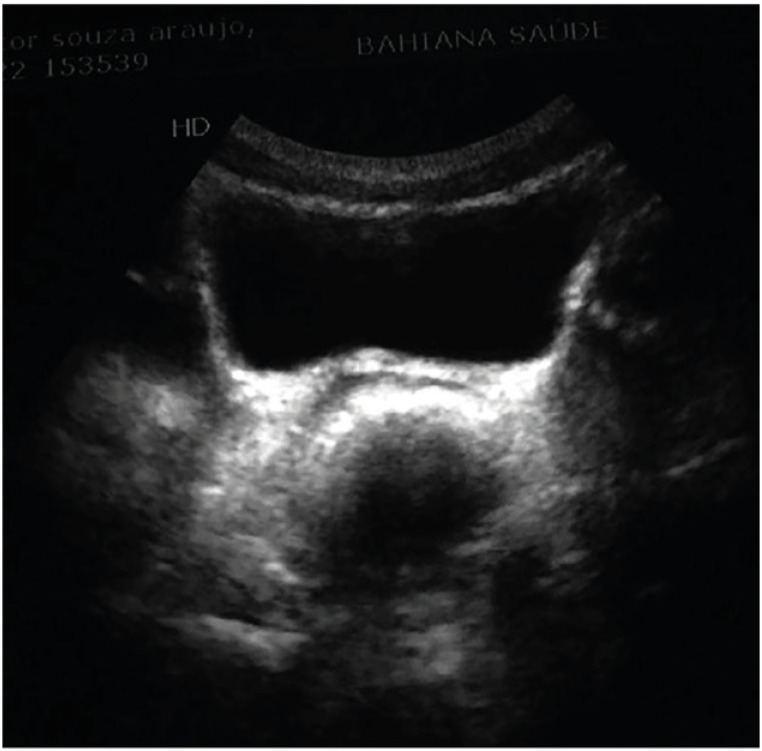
Increased rectal diameter in trans-abdominal ultrasound.
